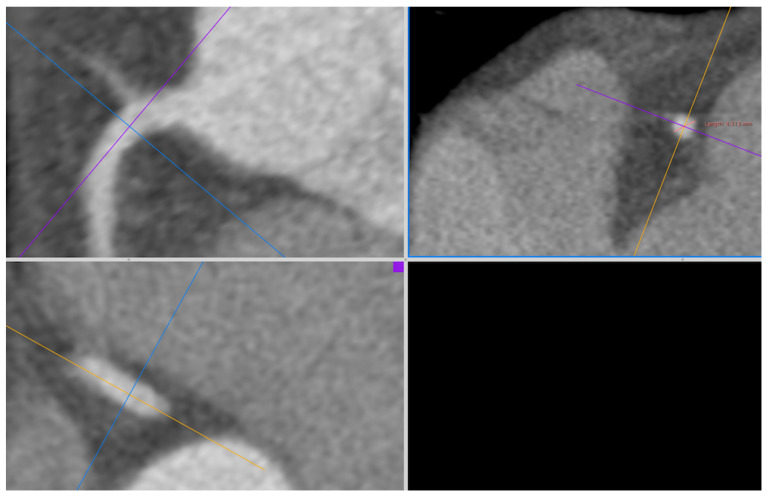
Three-dimensional curved MPR CT image depicting the measurement of RCA diameter; CT: computed tomography; MPR: multiplanar reformatted; RCA: right coronary artery.
